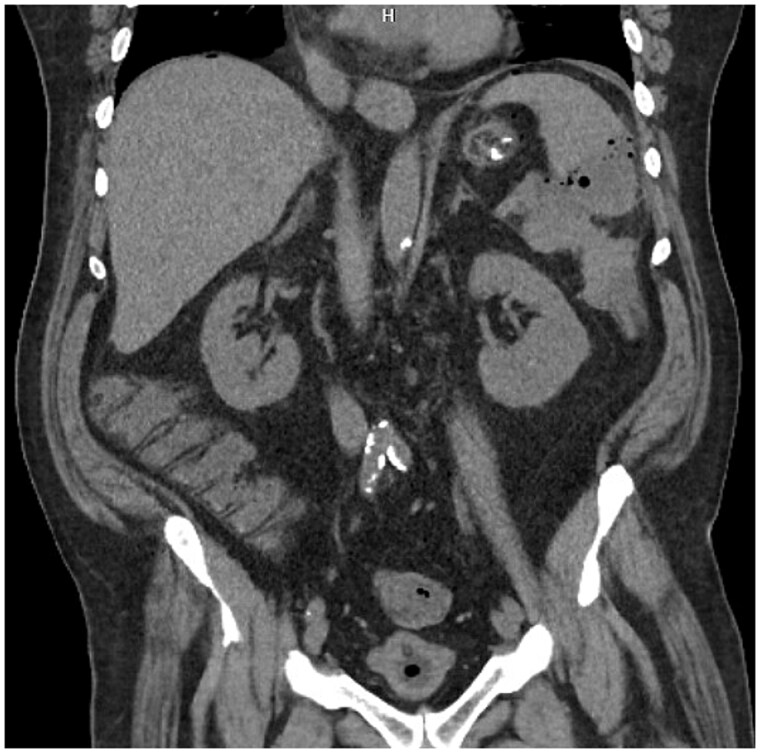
Coronal CT section demonstrating the splenic flexure mass adherent to the pancreatic tail and spleen.
